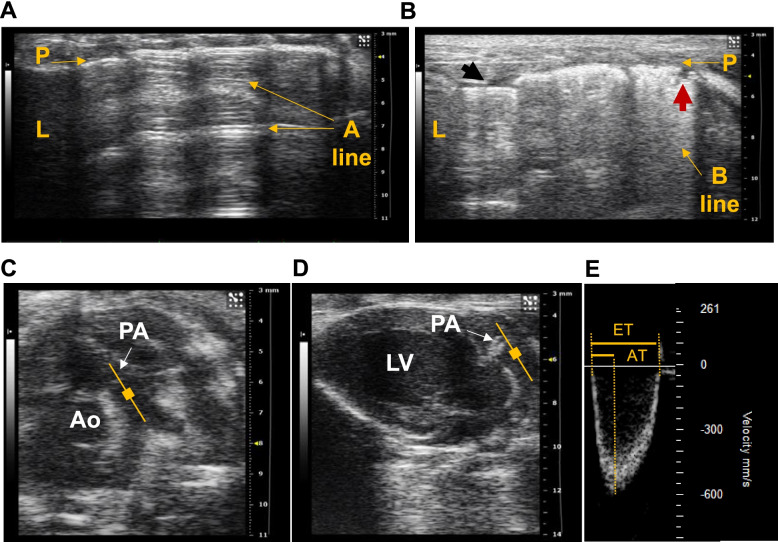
Lung and pulmonary artery evaluation by echocardiography. A Normal lung ultrasound (LUS), represented by the presence of A lines. B LUS showing broad B-lines indicating pulmonary oedema. The black arrow shows mild pleural effusion and the red arrow shows a disruption in the pleural surface, usually caused by increased hydrostatic pressure in lungs. C Short axis view at the level of the greater vessels and D a modified angle long axis view used to assess pulmonary artery flow. The yellow filled square indicates the sample volume position to obtain the flow using pulse wave Doppler. E Pulmonary artery flow with the ejection and acceleration time represented (ET and AT, respectively). P, pleural line; L, liver. Ao, aorta; PA, pulmonary artery valve
